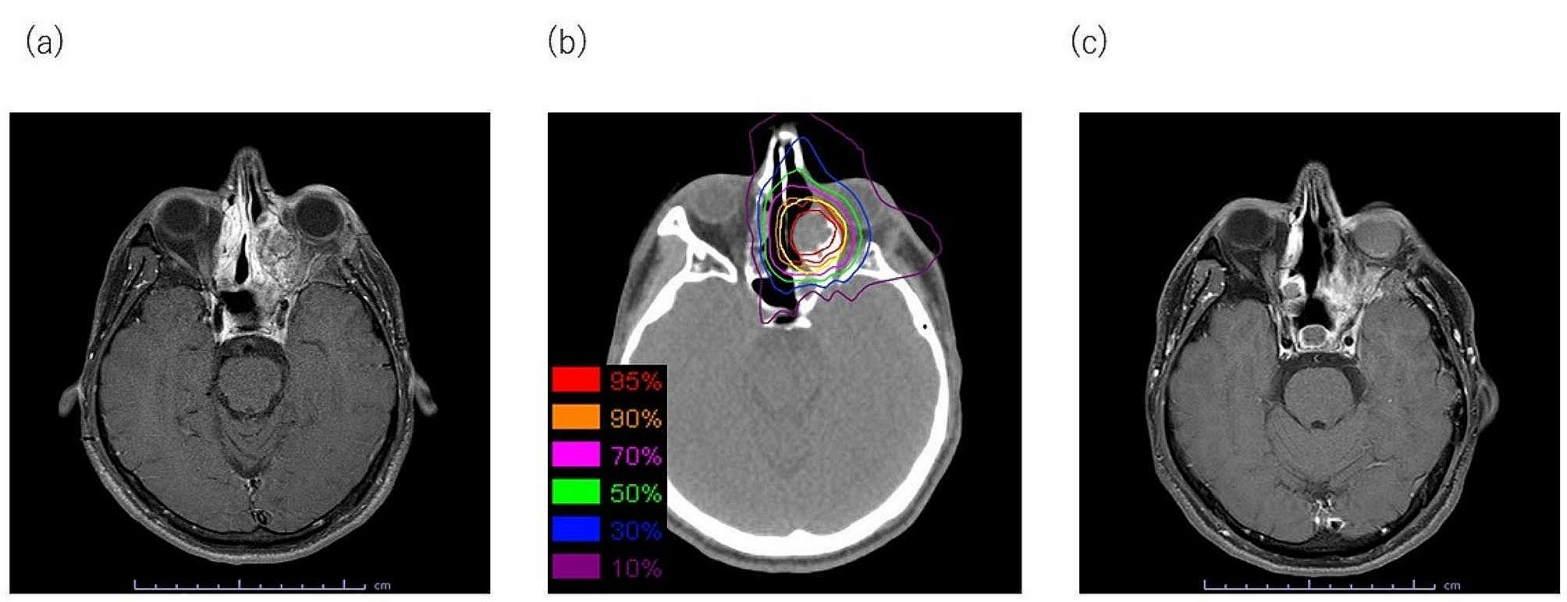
Re-irradiation using carbon-ion radiotherapy for recurrent maxillary clear cell odontogenic carcinoma (a) Nineteen months after carbon-ion radiotherapy: The tumor is enlarged and diagnosed as recurrent in axial, contrast-enhanced T1 weighted magnetic resonance imaging (MRI).(b) Dose distribution of re-carbon-ion radiotherapy, with a prescribed dose of 64 Gy in 16 fractions: The isodose lines correspond to 95%, 90%, 70%, 50%, 30%, and 10% dose areas. Representative computed tomography images are used for the delineation of gross tumor volume (red) and planning target volume (yellow) (c) Six years after re-carbon-ion radiotherapy: The tumor do not show growth or recurrence, and the patient has a stable disease in axial, contrast-enhanced T1 weighted MRI.
